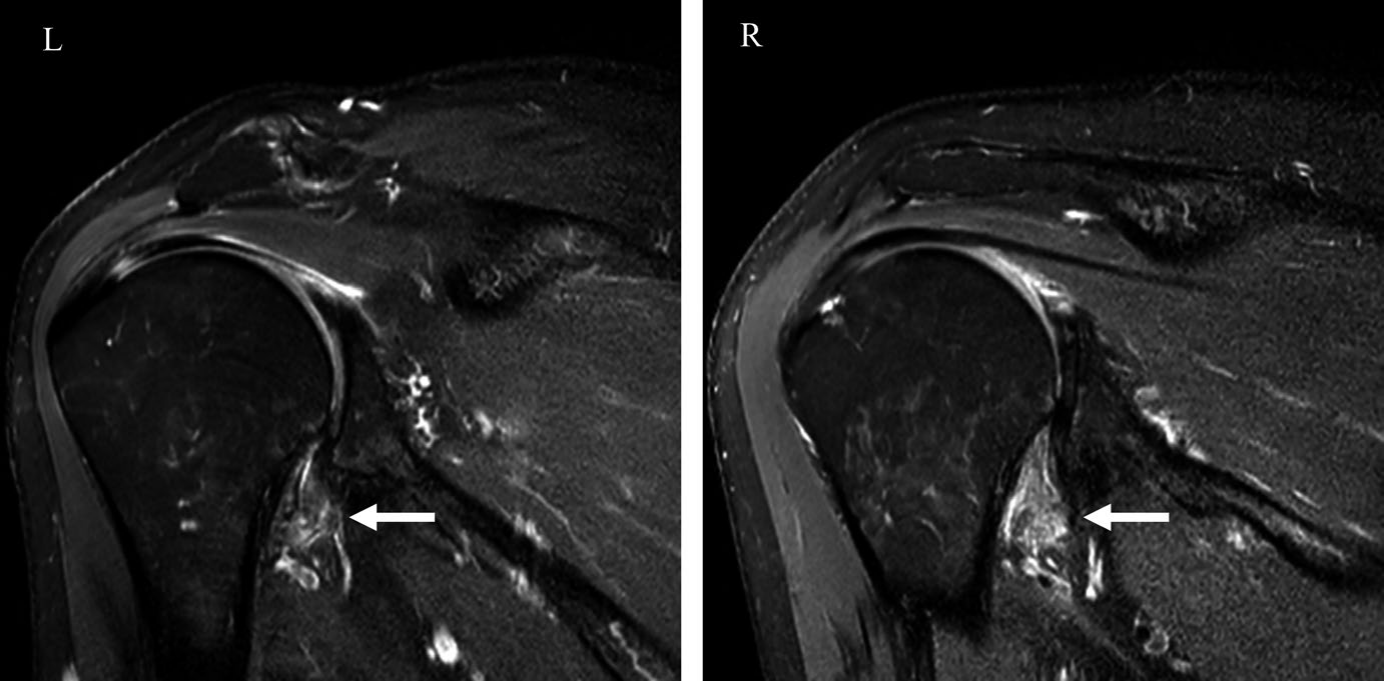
Magnetic resonance imaging of the bilateral shoulders depicting thickened and edematous inferior glenohumeral ligaments (white arrows). L, left; R, right
